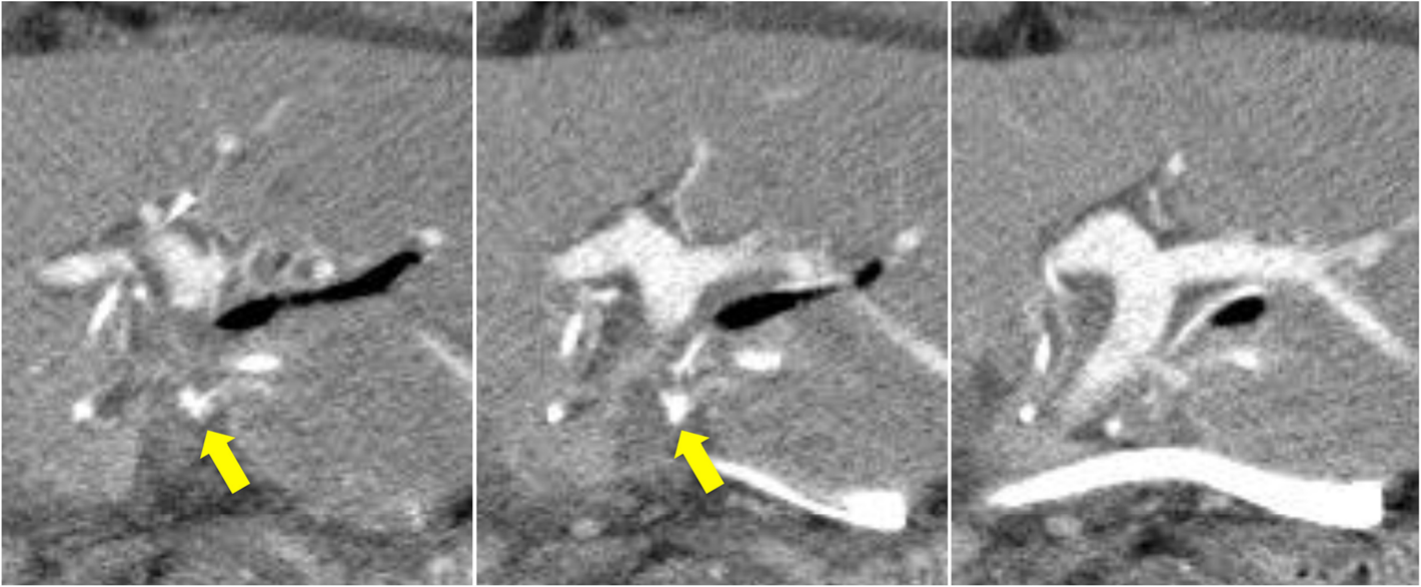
Computed tomography revealing a 6-mm aneurysm of the left hepatic artery on postoperative day 6
